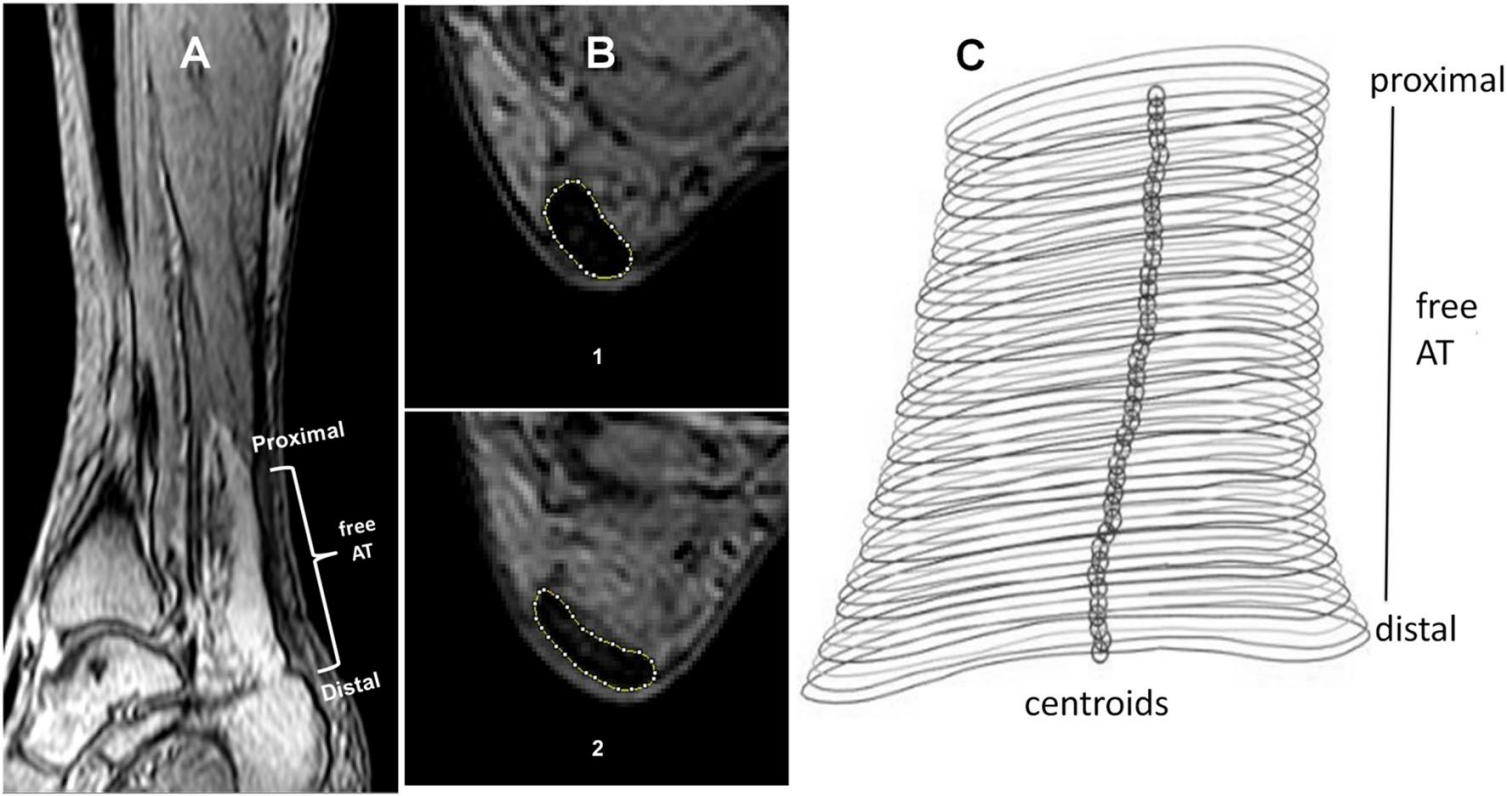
Magnetic resonance images and 3D contour plot of the free Achilles tendon (AT). Sagittal images (a) were used to determine the AT proximal and distal ends (M. soleus-AT junction, and AT attachment point on the calcaneum, respectively). Transversal images (b) between the AT proximal and distal ends were manually segmented to determine the AT cross-sectional area (CSA). Free AT length was determined as the curved path through the CSA centroids of each transversal slice (c)
